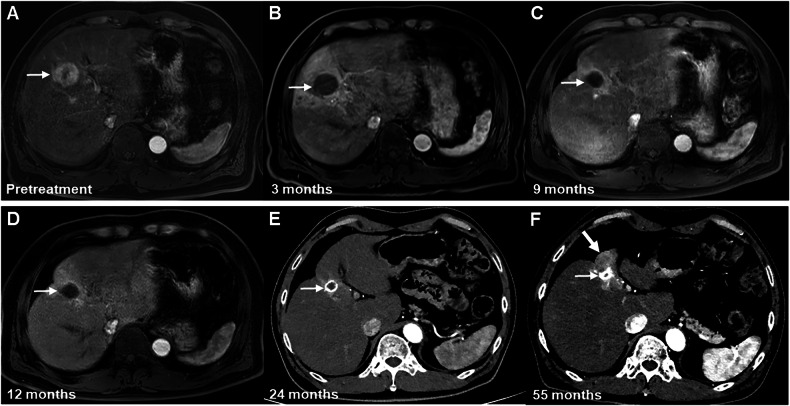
Images of a 61-year-old man with HCC treated by SIRT. MRI or CT obtained during the arterial phase. A Pretreatment contrast-enhanced MRI scan shows a 3.7 cm clinically diagnosed HCC (arrow) at segment 4 of the liver. B On the posttreatment contrast-enhanced MRI scan performed at 3 months after SIRT, no masslike enhancement was seen in the treated lesion (arrow) or along its margin; therefore, this lesion was assigned to the LR-TR nonviable category. C–E On 9–24-month follow-up CT or MRI scans, no masslike enhancement was seen in the treated lesion (arrows) or along its margin; instead, smooth perilesional enhancement was observed on (C) 9- and (D)12-month follow-up MRI scans, and smooth uniform calcification along the lesion (arrow) was observed on (E) 24-month follow-up CT scan. Hence, this lesion remained in the LR-TR nonviable category for a long period. F On 55-month follow-up CT scan, however, there was a 2.6 cm newly appeared masslike enhancement (thick arrow) along the margin of the treated lesion (thin arrow); thus, this lesion evolved into the LR-TR viable category. CT, computed tomography; HCC, hepatocellular carcinoma; LR, liver imaging reporting and data system; MRI, magnetic resonance imaging; SIRT, selective internal radiation therapy; TR, treatment response
